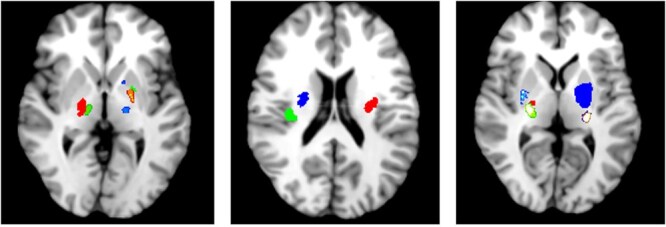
Axial magnetic resonance imaging (MRI) sample distribution of lacunar infarct location in our study population.
